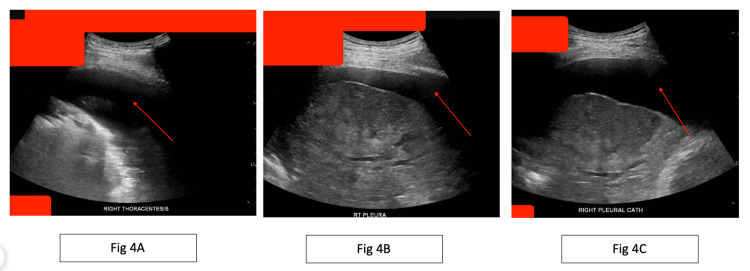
Multiple chest ultrasounds during the patient’s hospital stay. Pleural effusion can be seen reaccumulating, requiring multiple thoracenteses, all on the right side (A-C).
